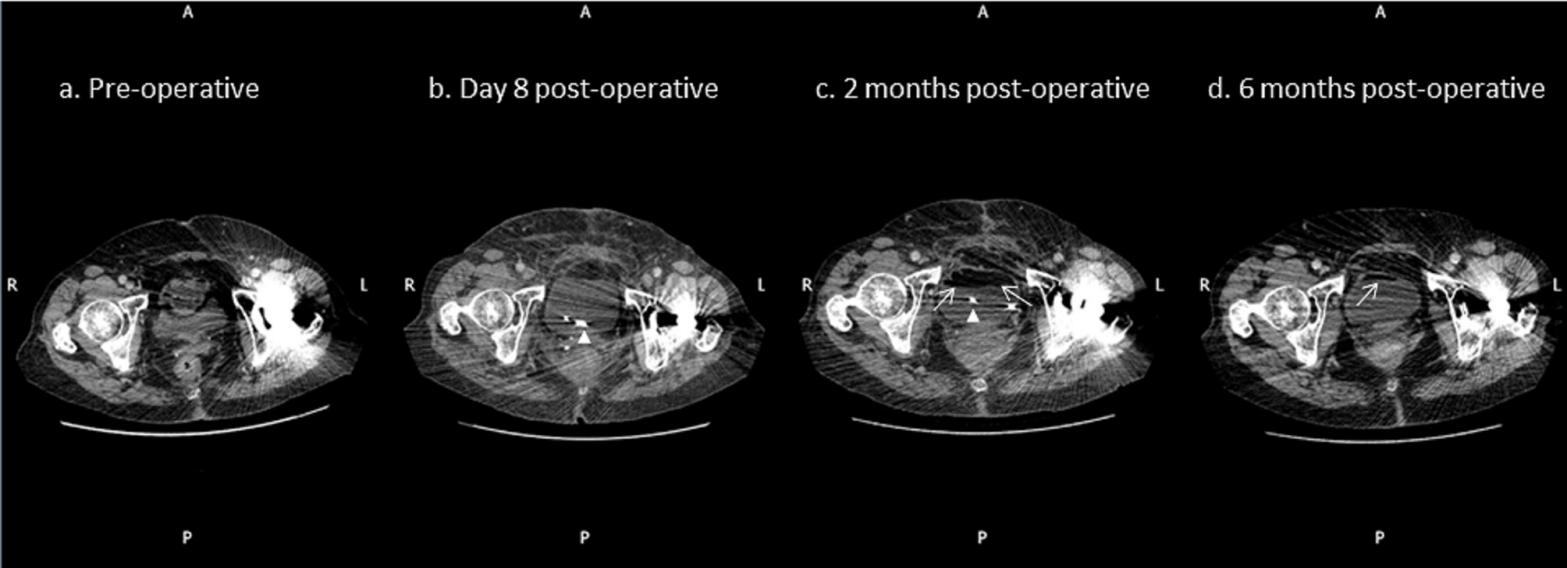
(a) Axial CT images through the pelvis at varying time points (pre-operative; 8 days, 2 months, and 6 months post-operative) demonstrating absence of fat/fluid level within the bladder (b).subsequent presence of intravesical fat as indicated by red arrowheads (c). and reduced volume at 6 months post-operative (d). (The patient’s ureteric stent has been removed).
